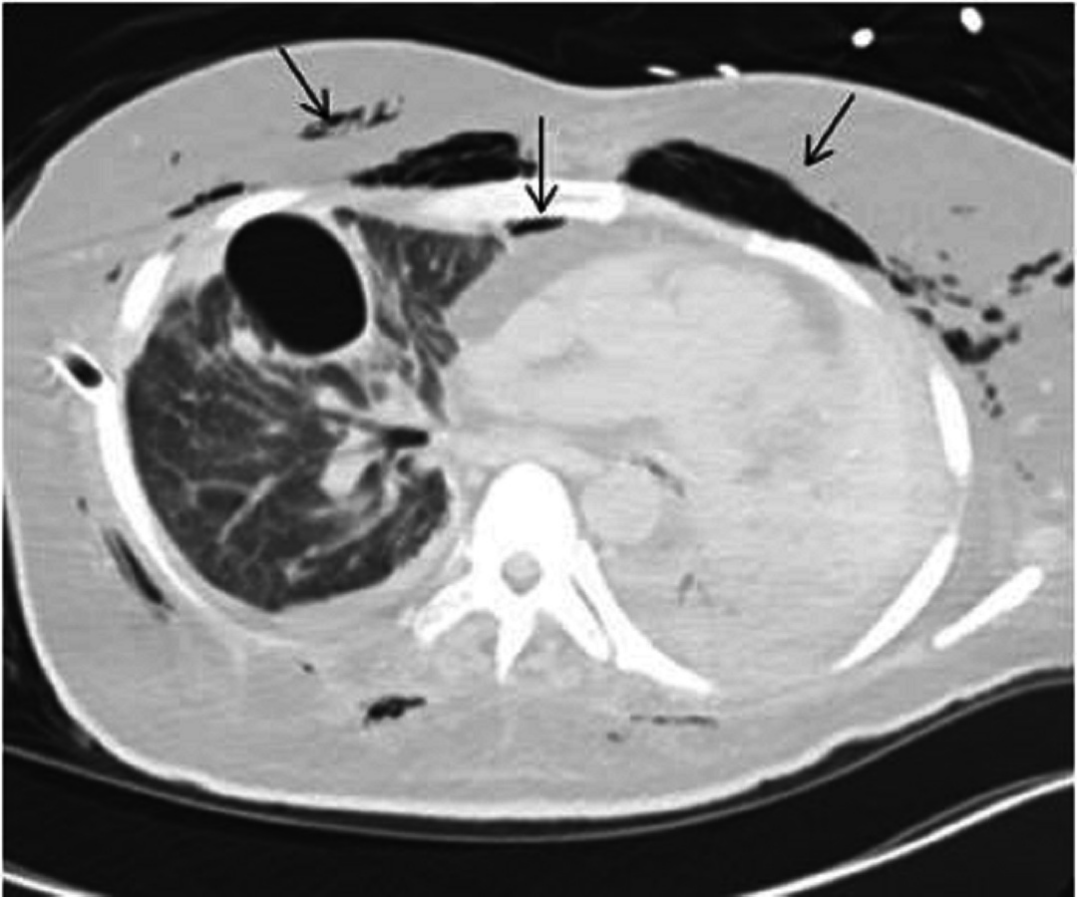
Subcutaneous emphysema and pneumomediastinum on computed tomography (CT) of the chest as indicated by arrows
